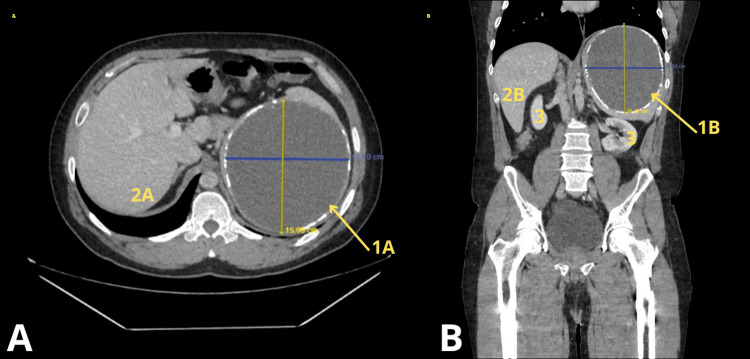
Computed tomography. Axial (A) and coronal (B) abdominal CT images demonstrating a primary splenic cyst. 1A: Axial view showing the splenic cyst. 2B: Coronal view showing the splenic cyst. 2A: Axial view of the liver. 2B: Coronal view of the liver. 3: Coronal view showing the right and left kidneys.
